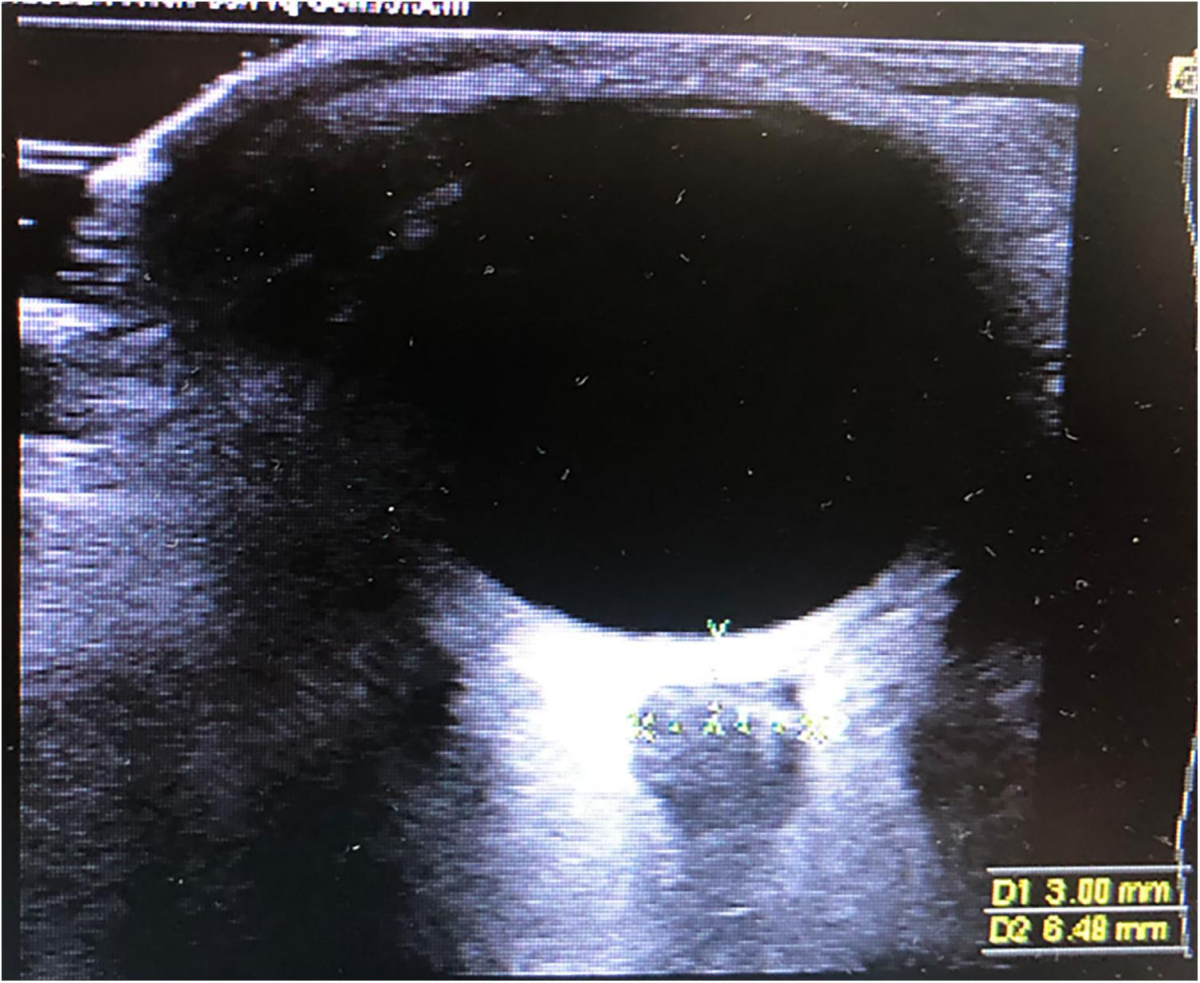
Ultrasonographic OSND measurement.
